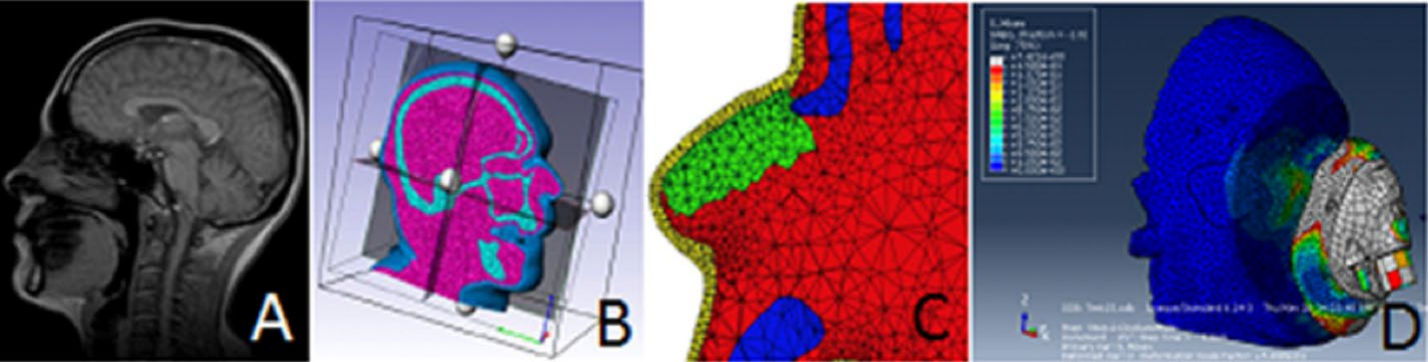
Conversion of CT stacked images (A) into a mesh containing tetrahedral elements (B). C Material properties are assigned to the model including skin (yellow), bone (blue), cartilage (green) and muscle (red). D Von Mises stress on the skin and medical device (respiratory mask)
